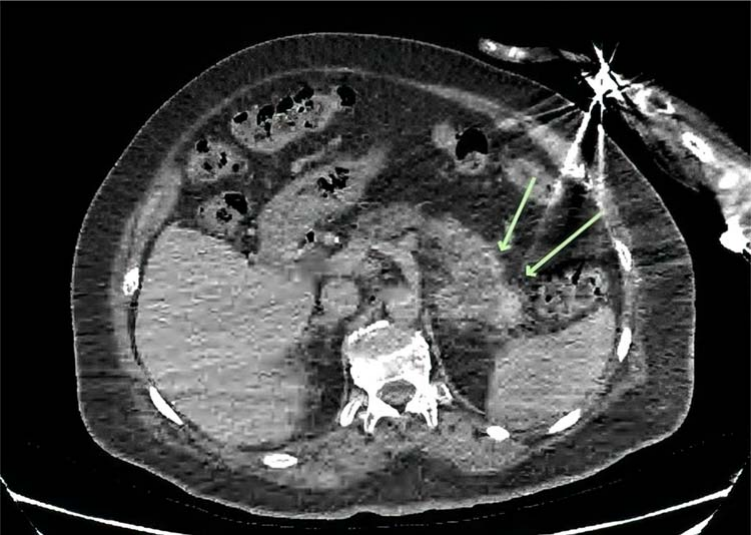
Axial CT of the abdomen demonstrating a necrotic mass centred in the tail of the pancreas measuring 57 × 43 mm (green arrows).
